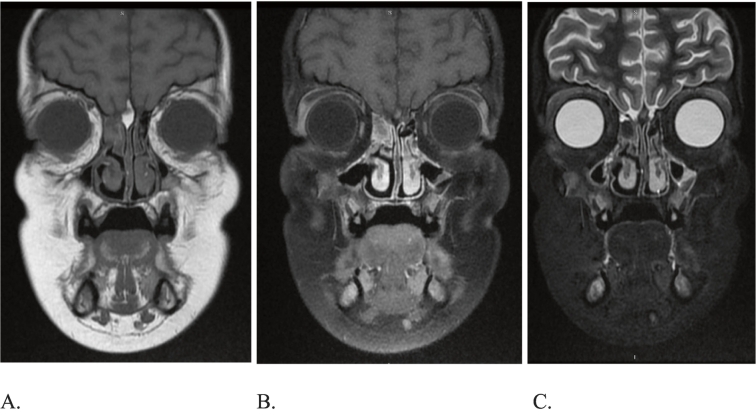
Right anterior ethmoidal sinus lesion on coronal view of sinus MRI. A: Low signal intensity on T1. B: No significant enhancement on T1 with contrast and fat suppression. C: Low signal intensity on T2.
